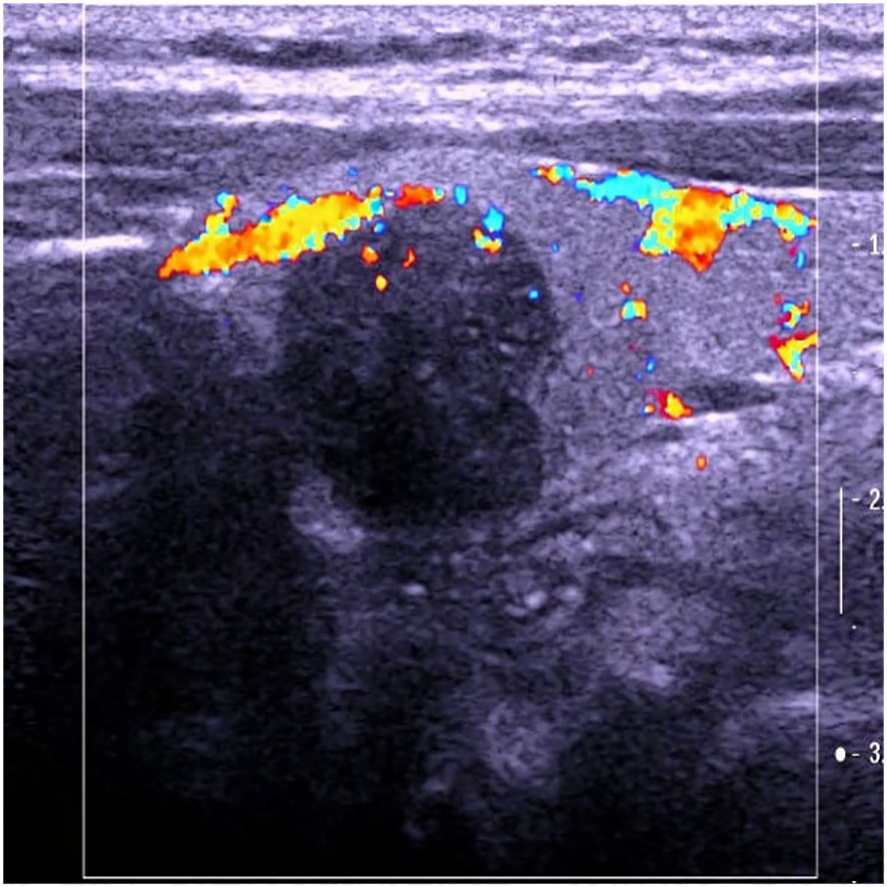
Color Doppler of atypical parathyroid adenoma misdiagnosed as “thyroid malignant tumor”.
